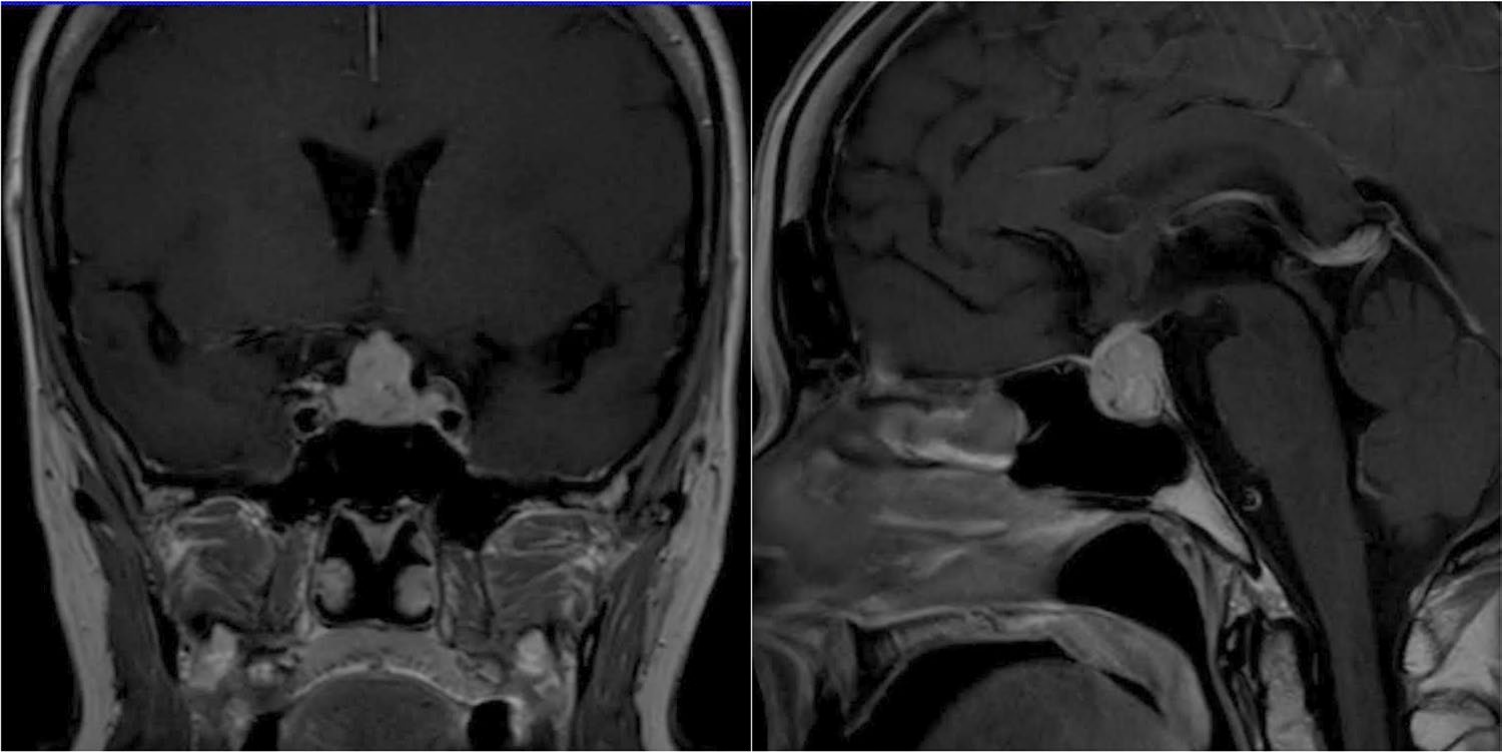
Coronal (left) and sagittal (right) pre-operative MRI scans showing the inhomogeneous sellar lesion with suprasellar extension. *MRI* magnetic resonance imaging
